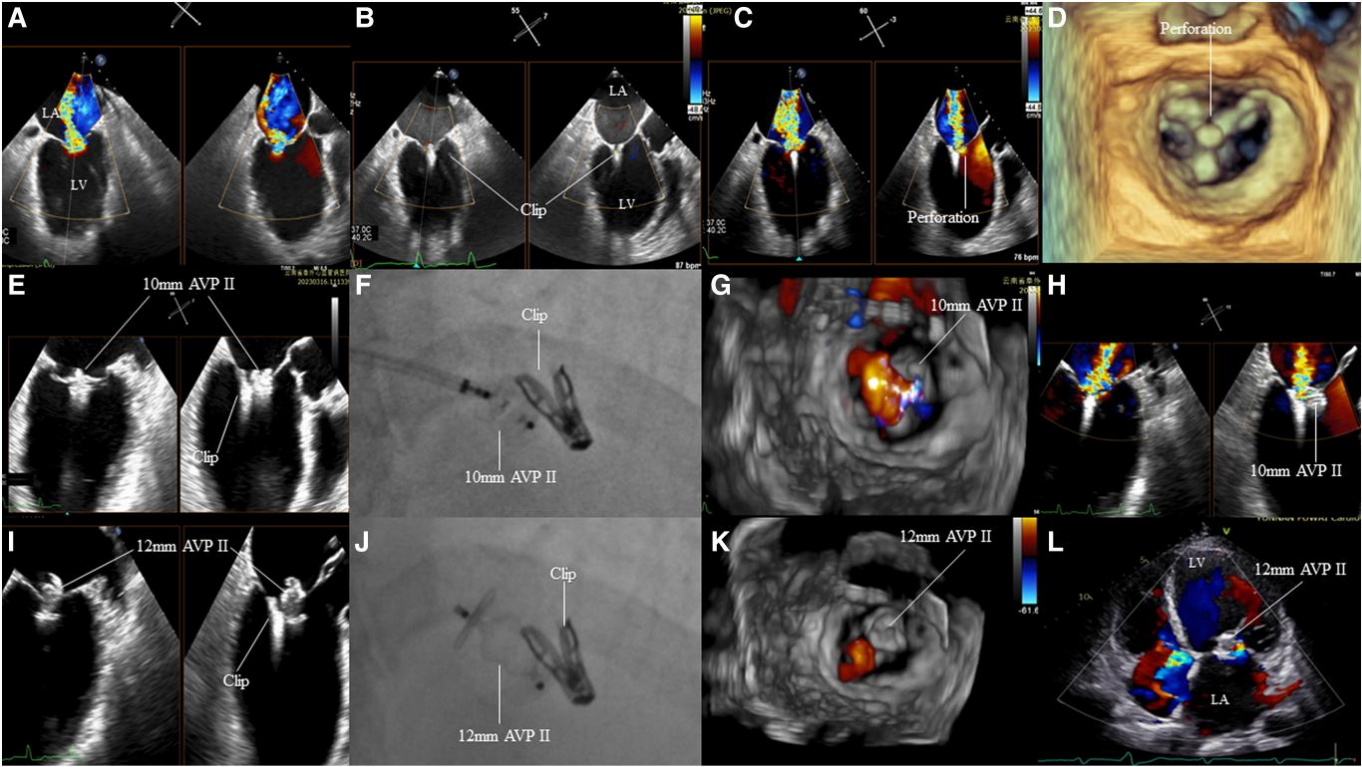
(*A*–*D*) Recurrent mitral regurgitation (MR) was noted due to anterior leaflet tear. (*E*–*H*) Suboptimal MR reduction and occluder morphology were noted due to insufficient oversize [10 mm Amplatzer Vascular Plug (AVP) device]. (*I*–*K*) After using 12 mm AVP with sufficient oversize, MR was reduced to 1+ to 2+. (*L*) Transoesophageal echocardiography in 1-year follow-up revealed 1+ MR.
